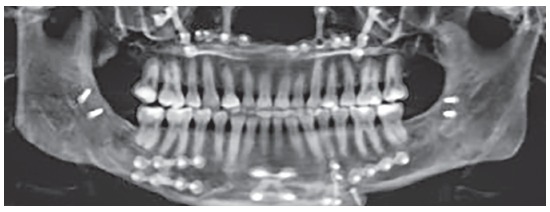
Panoramic radiograph after 1-year postoperative follow-up.
